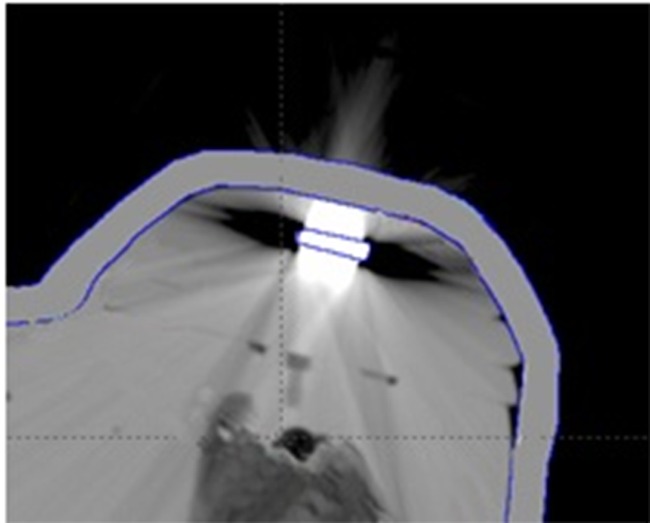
CT image showing artifacts due to the presence of the magnetic disk.
